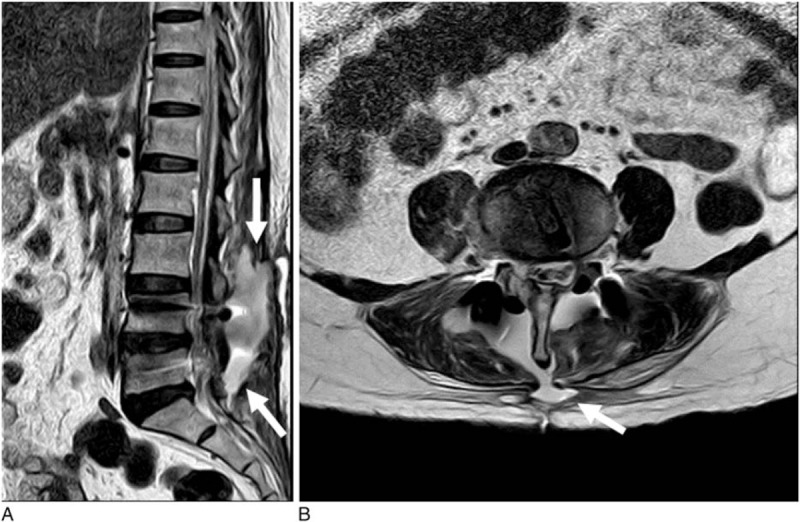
Lumbosacral MRI performed on the 18th day after posterior lumbar and interbody fusion at L4–L5. (A) Sagittal T2-weighted image showing a large amount of cerebrospinal fluid (arrows) in the lumbosacral space. (B) Axial T2-weighted MRI showing a cerebrospinal fluid cutaneous fistula (arrow). MRI = magnetic resonance imaging.
